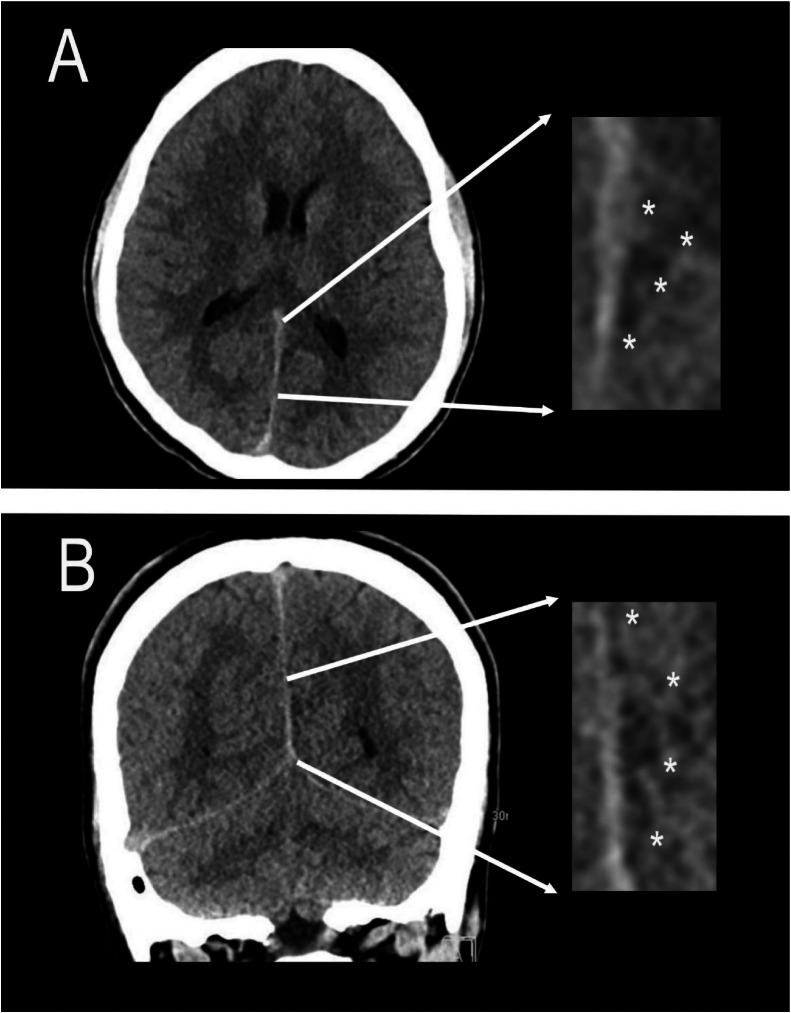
Axial noncontrast CT of the head (A) and Coronal noncontrast CT of the head (B) each with blown up field of view in the region of interest showing a very subtle triangular hypodensity in the medial left occipital lobe abutting the falx (demarcated by white asterisks) overlooked on initial imaging.
